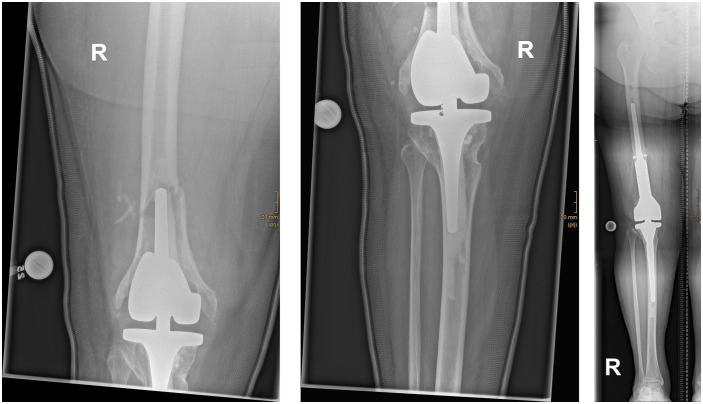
Example radiographs of a trauma patient: (a) preoperative distal femur, (b) preoperative proximal tibia, and (c) postoperative radiograph following distal femoral replacement.
